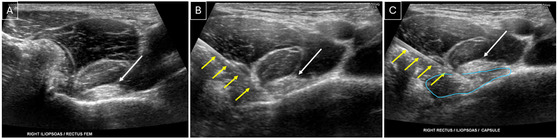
Iliopsoas bursa injection. Transverse ultrasound image of the iliopsoas tendon (solid white arrow) at the level of the pelvic brim (A). The needle (solid yellow arrows) is advanced in plane to the transducer until it is positioned on bone just lateral to the tendon within the potential space of the iliopsoas bursa (B). The therapeutic anesthetic and steroid mixture is then injected, distending the bursa (blue outline, C).
